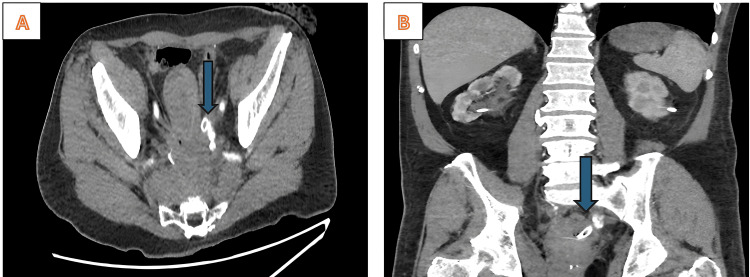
CT angiography demonstrating left internal iliac artery aneurysm adjacent to a ureteric stent A: Axial image showing a saccular aneurysm of the left internal iliac artery (arrow) closely abutting the distal ureteric stent; B: Coronal reconstruction confirms the aneurysm’s proximity to the distal left ureter (arrow), consistent with an arterio-ureteral fistula.
